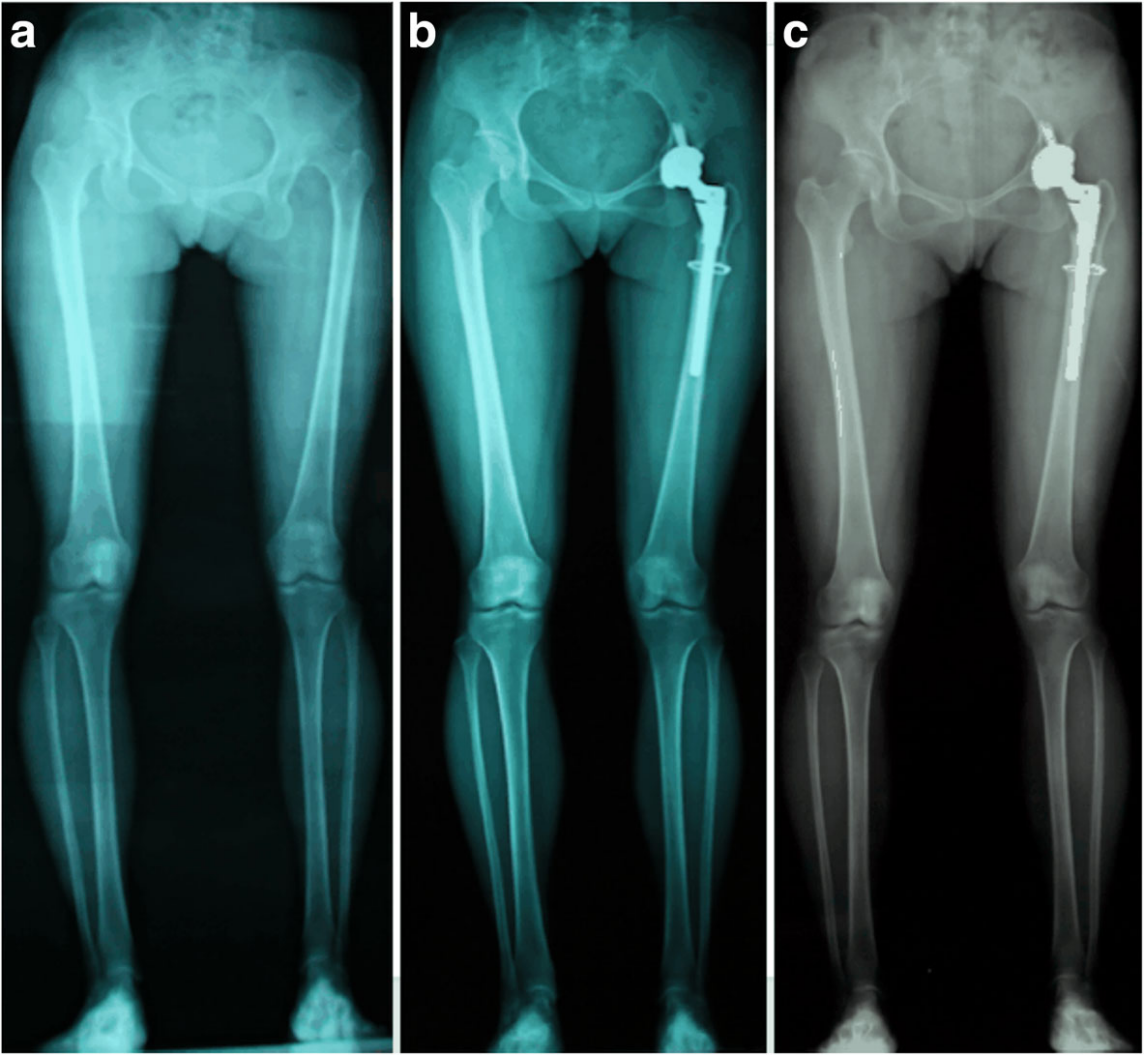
Full-length radiographs of a twenty-five-year-old woman with unilateral Crowe type-IV DDH. The serial post-operative radiographic image showed that pelvic inclination was normalized over time. (a) Pre-operative radiographic image; (b) Radiographic image after four years follow-up; (c) Radiographic image after nine years follow-up
